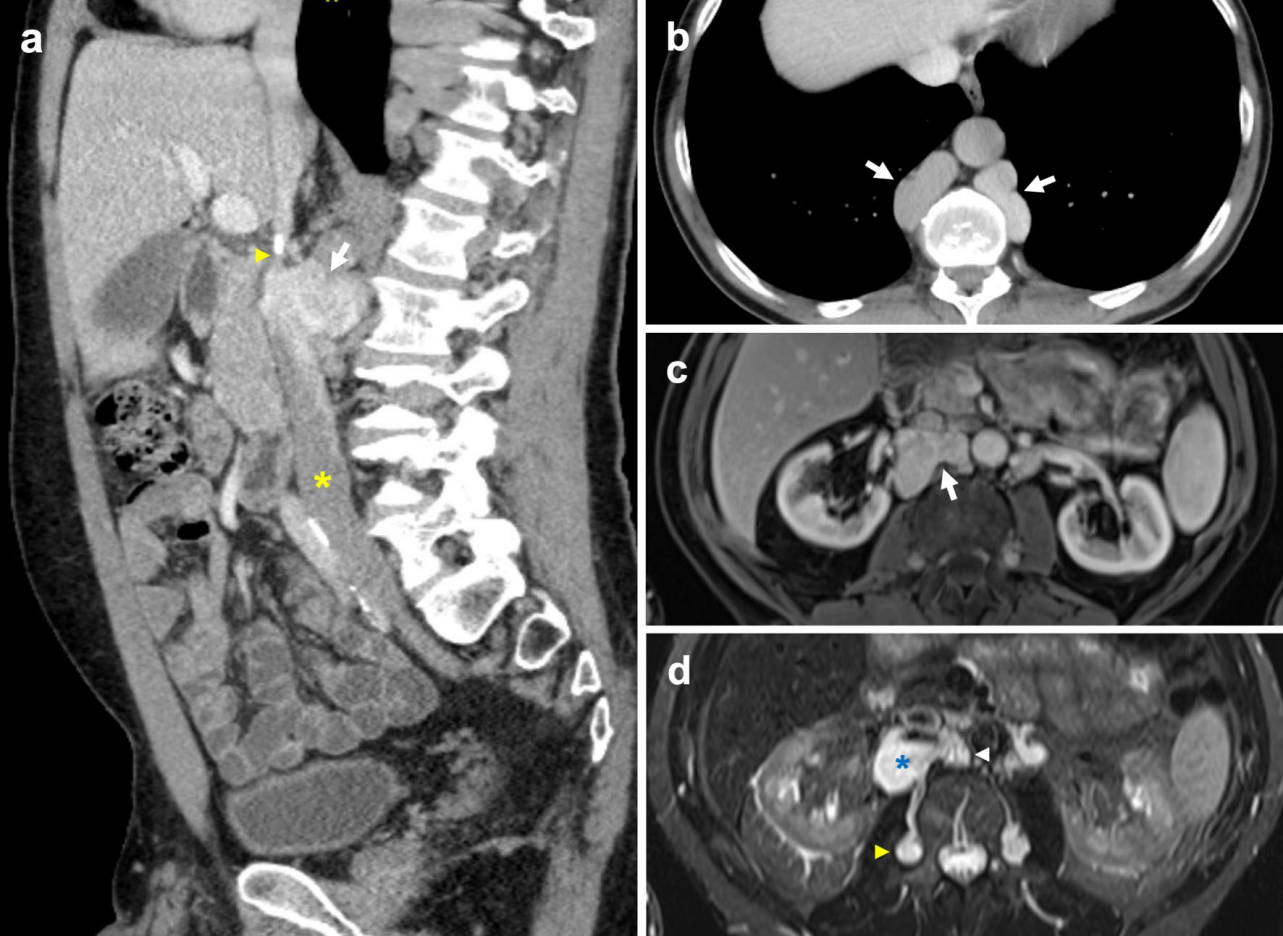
Distended collateral vein mistaken for mass in context of incidentally detected suprarenal IVC stenosis. The 54-year-old man had no previous history of IVC filter insertion or intrabdominal surgery. a Contrast-enhanced sagittal CT shows a pseudomass (arrow) immediately posteroinferior to complete stenosis (arrowhead) of the suprarenal IVC, with caudal segments of the IVC (asterisk) preserved. A small focus of calcification is present at the level of the stenosis. b Axial CT shows preferential blood flow through markedly distended azygos and hemiazygos veins (arrows). c Axial MR T1 VIBE image shows a well-defined lobulated mass (arrow) with venous enhancement. d Axial MR T2 image of the mass (asterisk) shows high T2 signal in keeping with slow venous flow. It communicates posteriorly with the right ascending lumbar vein (yellow arrowhead) and medially with a right paravertebral collateral vein (white arrowhead)
